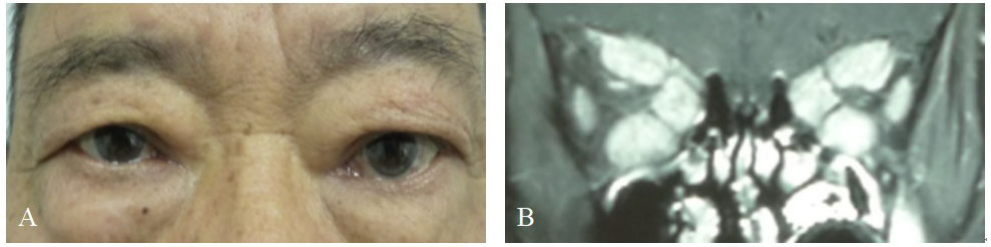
Representative examples of thyroid-associated ophthalmopathy (TAO) patients only with extraocular muscles involvement. (A) TAO patients without upper eyelid retraction and conjunctival congestion, chemosis, and prominent eyes. (B) Coronal enhanced MRI scans showing thickening and infammation of the superior, inferior and medial rectus muscle of the bilateral eye.
